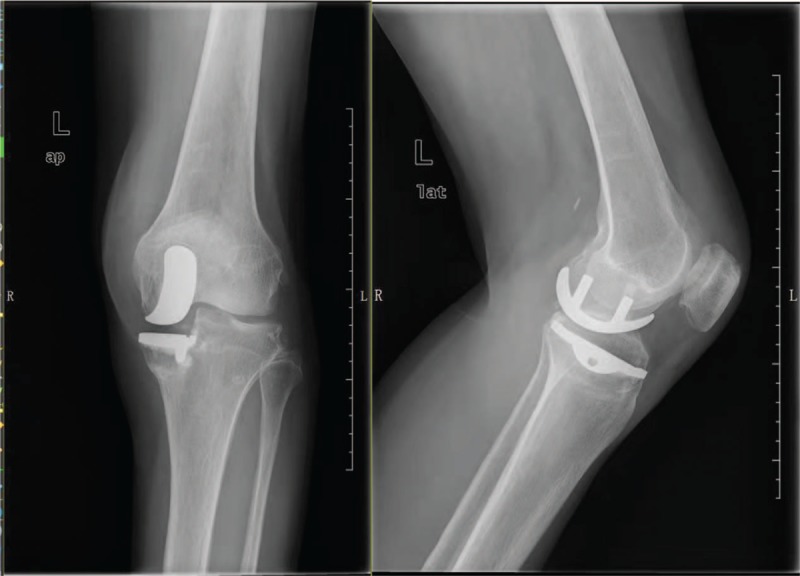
X-rays showing medial malleolus fracture of the left knee.
